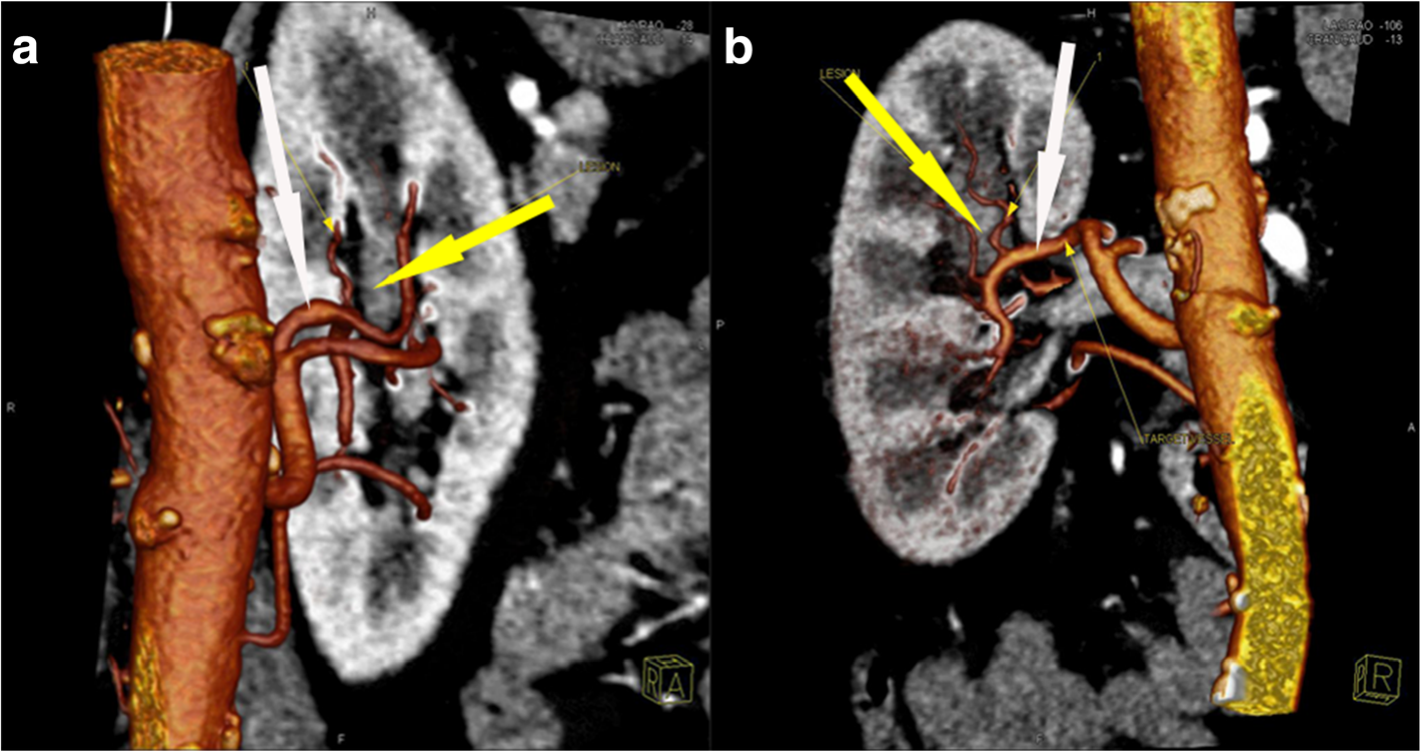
Computed tomography angiography showing the blood supply vessels (*white arrow*) of tumor region (*yellow arrow*) (a) Anterior view (b) Right view
